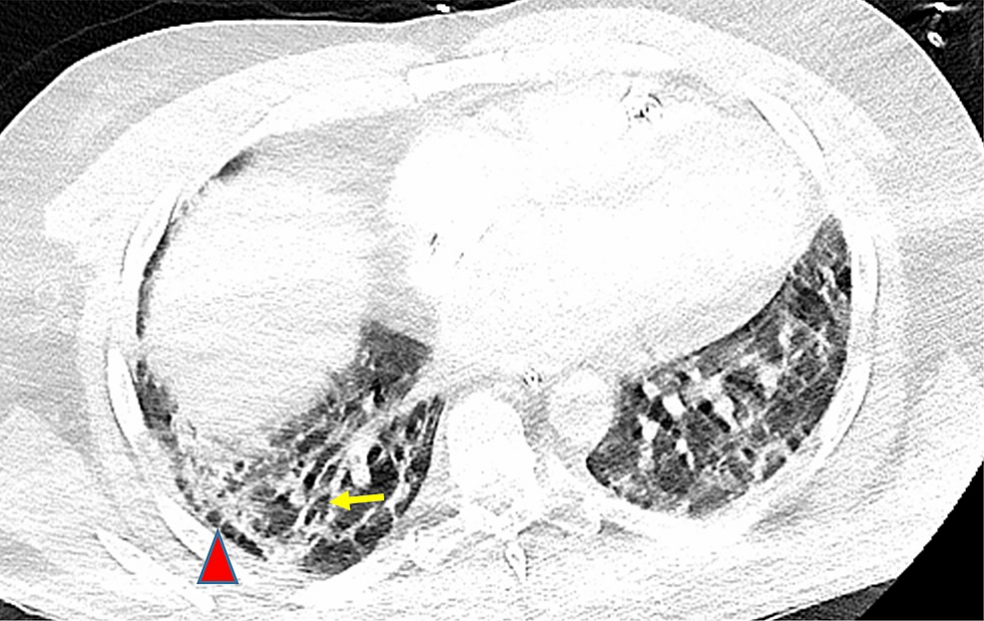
CT scan of the chest with contrast (axial image) shows traction bronchiectasis (yellow arrow) and subpleural reticulations (red arrowhead) in the right lung base that is suggestive of progressive fibrosis
